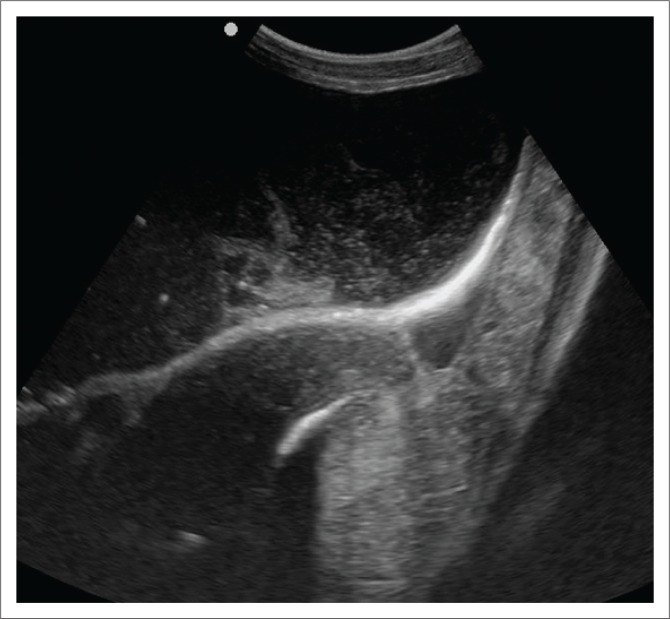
An ultrasonographic evaluation of the steenbok abdomen at presentation revealing distension of the bladder.
